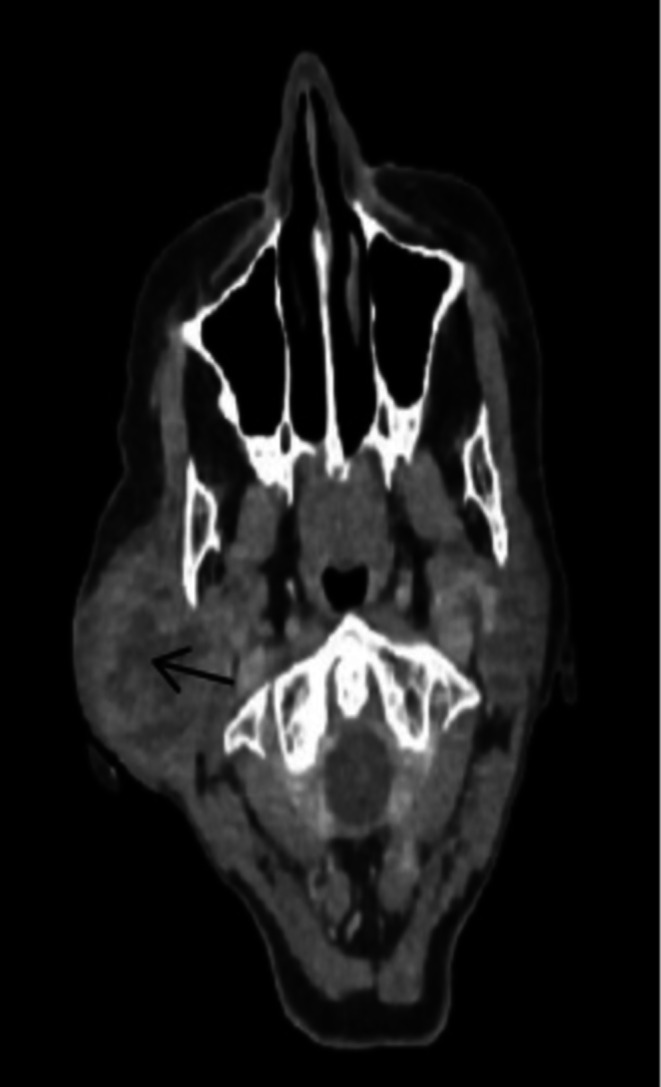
CT scan revealing an ill‐defined, irregular, multilobulated heterogeneous mixed‐density lesion originating from the right parotid gland.
